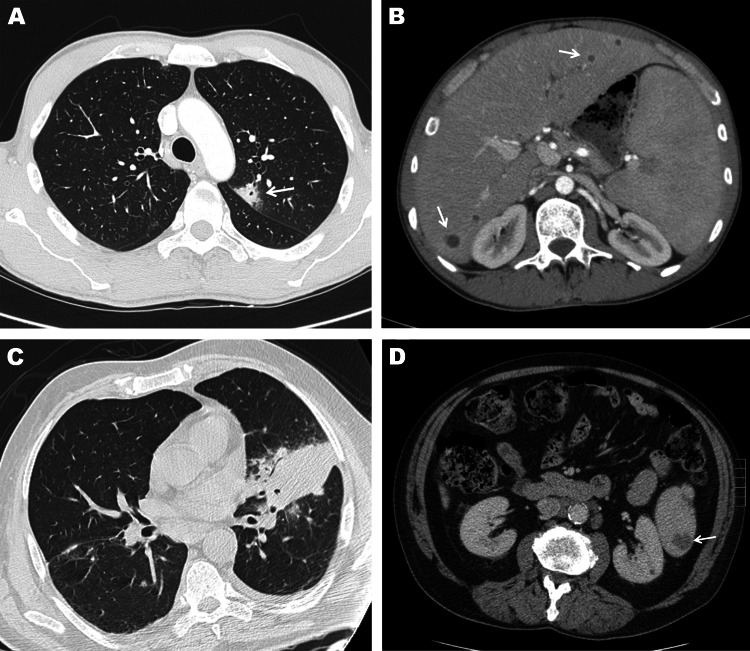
Computed tomography (CT) images of the chest and abdomen of case-patient 1 showing A) a subpleural nodular and cavitary lesion (arrow) in the left upper lobe of the lung and B) multiple small round liver abscesses, seen as multiple foci of ill-defined areas of hypoattenuation (arrows), and enlargement of the spleen. CT images of the chest and abdomen of case-patient 2 showing C) a focal area of parenchymal consolidation in the left lung associated with an ipsilateral mild pleural effusion and D) and a spleen abscess (arrow).
